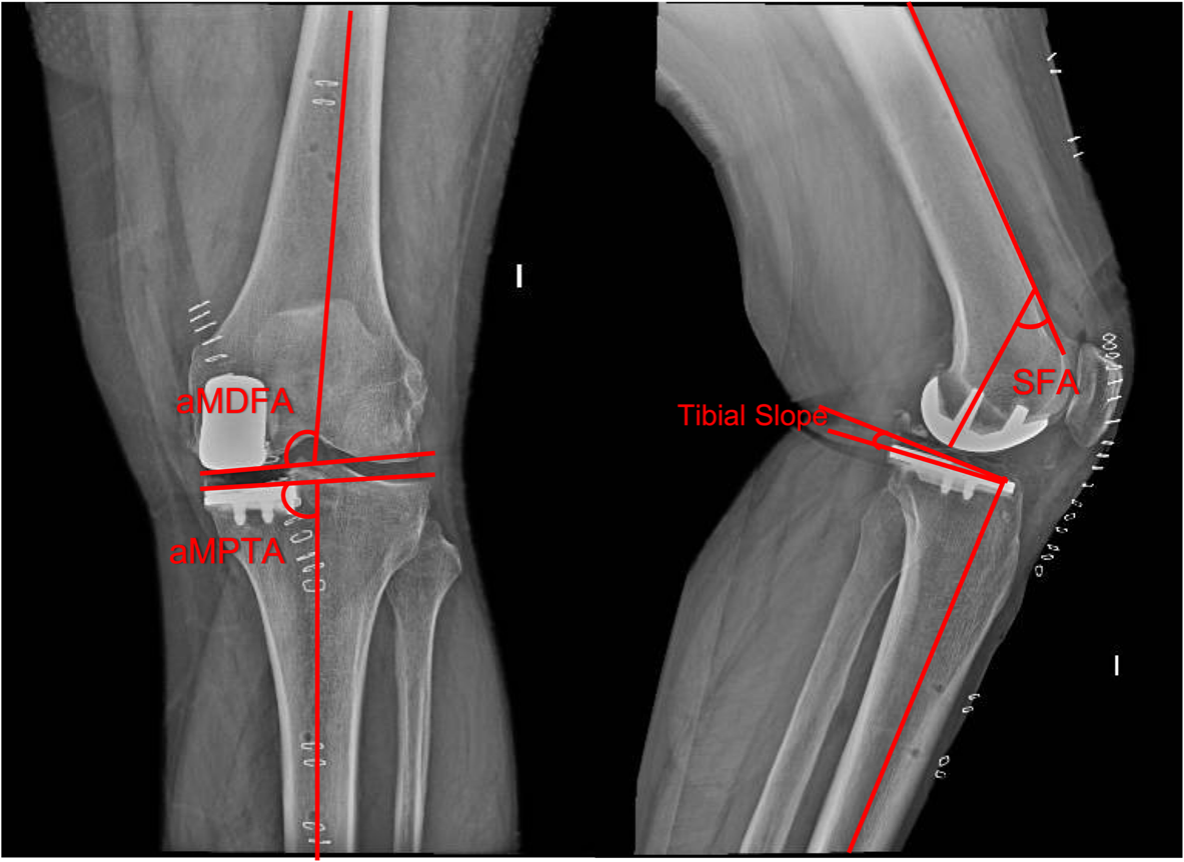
Radiological measures in the anteroposterior and lateral knee x-ray. *aMDFA* anatomical medial distal femoral angle, *aMPTA* anatomical medial proxial tibial angle, SFA sagital femoral angle
